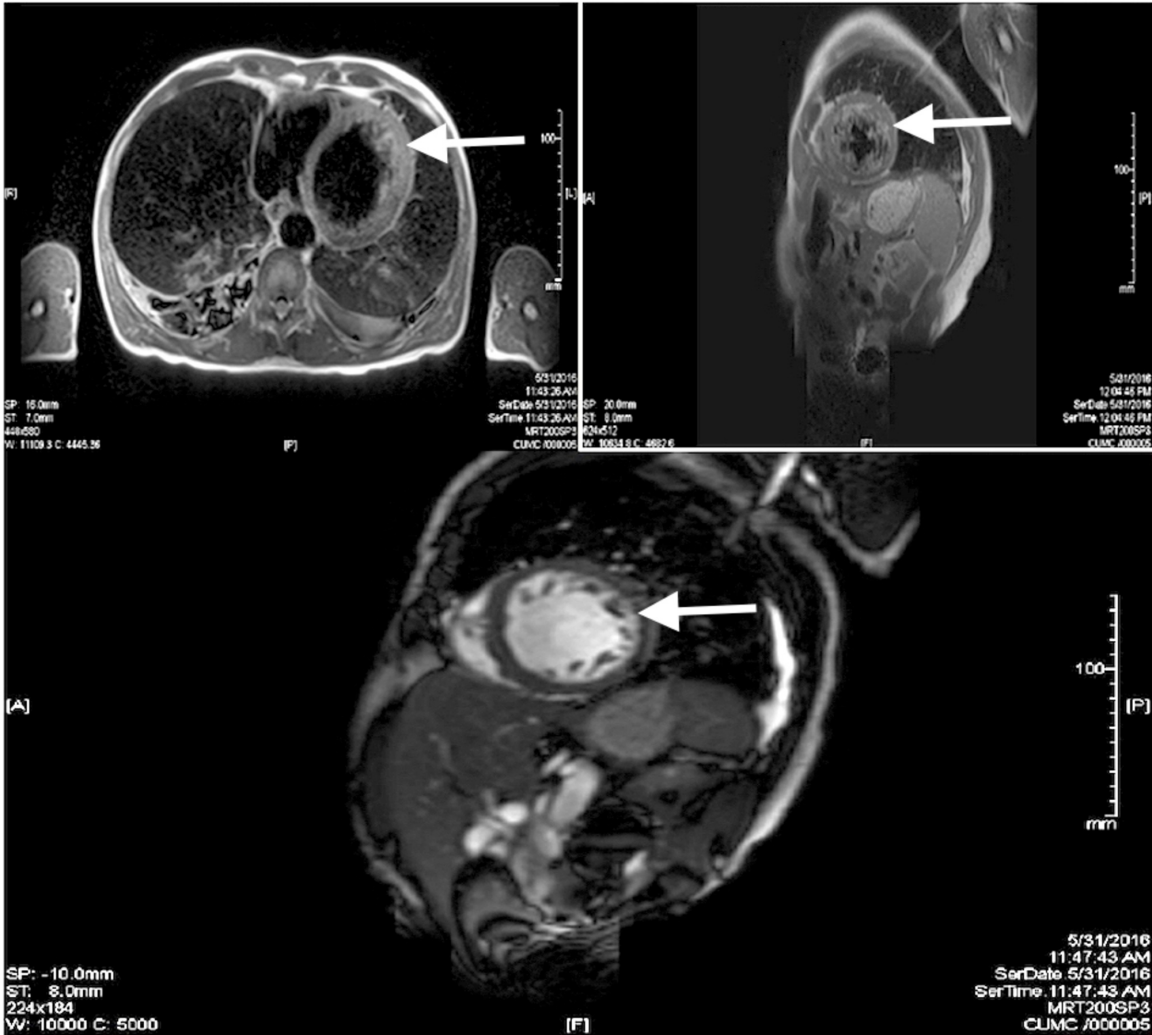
Cardiac MRI showing hyper-trabeculations (white arrows), assisting in the diagnosis of LVNC MRI: magnetic resonance imaging
